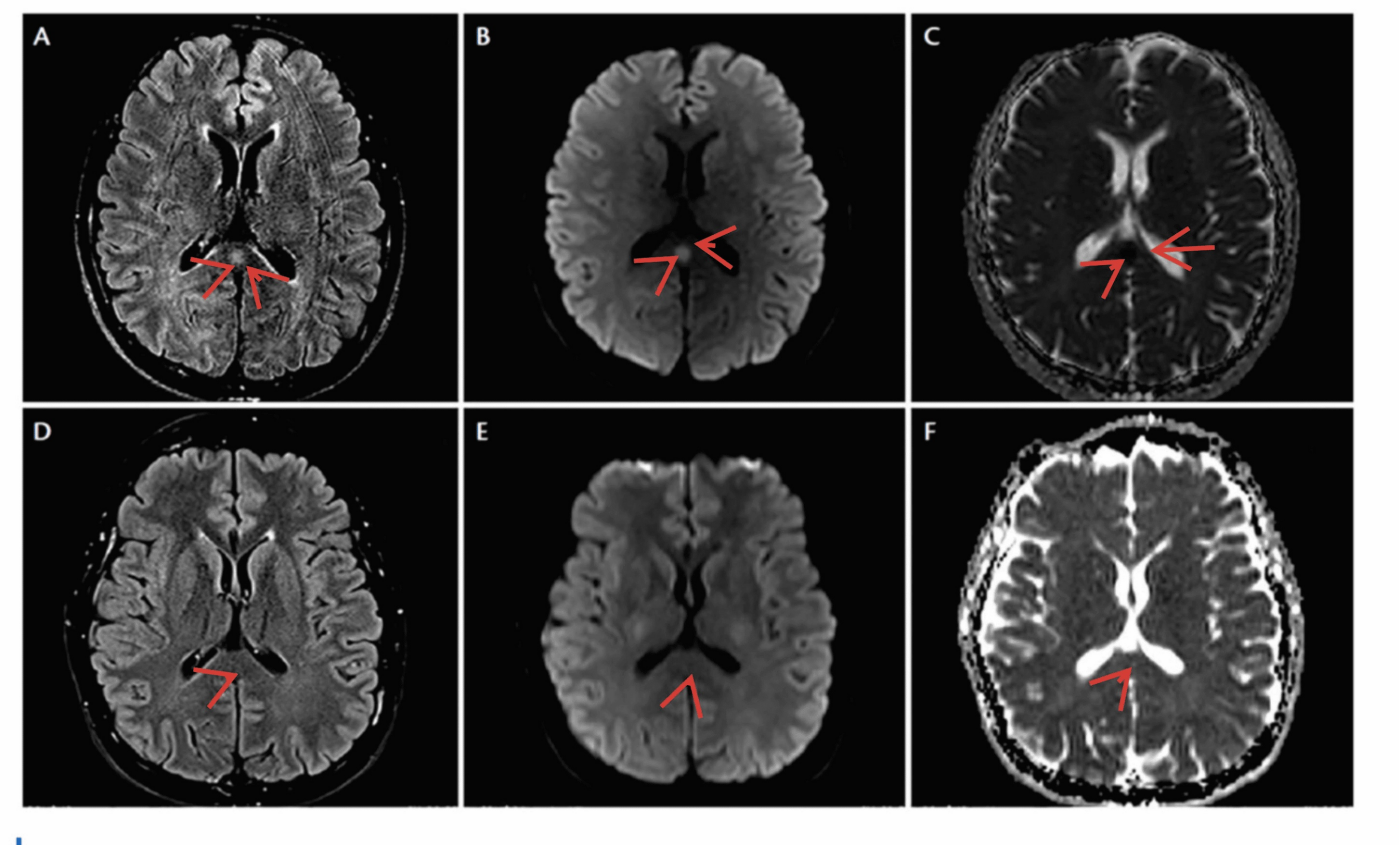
MRI of the head Brain MRI shows a small, ovoid area of increased signal intensity in the center of the splenium of the corpus callosum on axial FLAIR (A) and DWI (B), with corresponding diffusion restriction on the ADC image (C). Repeat FLAIR (D), DWI (E), and ADC (F) imaging performed at three months demonstrate complete resolution of the previously noted abnormal signals in the splenium. FLAIR, fluid-attenuated inversion recovery; DWI, diffusion-weighted imaging; ADC, apparent diffusion coefficient
